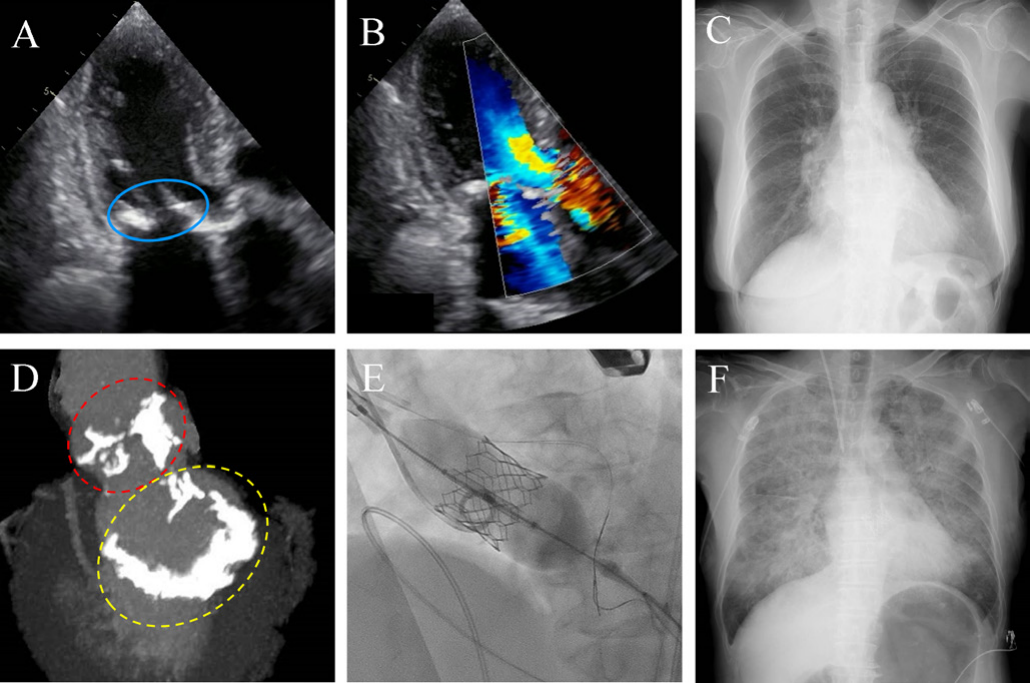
A representative case. (A): Preprocedural TTE image (parasternal long-axis view) LVEF was 63%, mean AVPG was 49 mm Hg, and AVAi was 0.41 cm^2^. The light blue circle indicates MAC. (B): Preprocedural TTE image (parasternal short-axis view). Restricted opening due to MAC. The mean AVPG was 15 mm Hg. The light blue circle indicates MAC. (C): Chest X-ray image before TAVID: Preprocedural MSCT findings. The red-dot circle indicates the aortic valve complex calcium with volume of 259 mm^3^. The yellow circle indicates the mitral valve calcification with a volume of 1923 mm^3^. (E): Transfemoral TAVI Implantation of a 23 mm Sapien three decreased the mean AVPG from 63 mm Hg to 9 mm Hg. (F): Chest X-ray image 2 hours after TAVI. The patient had shortness of breath and was diagnosed with development of ADHF. AVAi, aortic valve area index; ADHF, acute decompensated heart failure; AVPG, aortic valve pressure gradient; LVEF, left ventricular ejection fraction; MAC, mitral annular calcification; MSCT; multislice CT; TAVI, transcatheter aortic valve implantation; TTE, transthoracic echocardiography.
